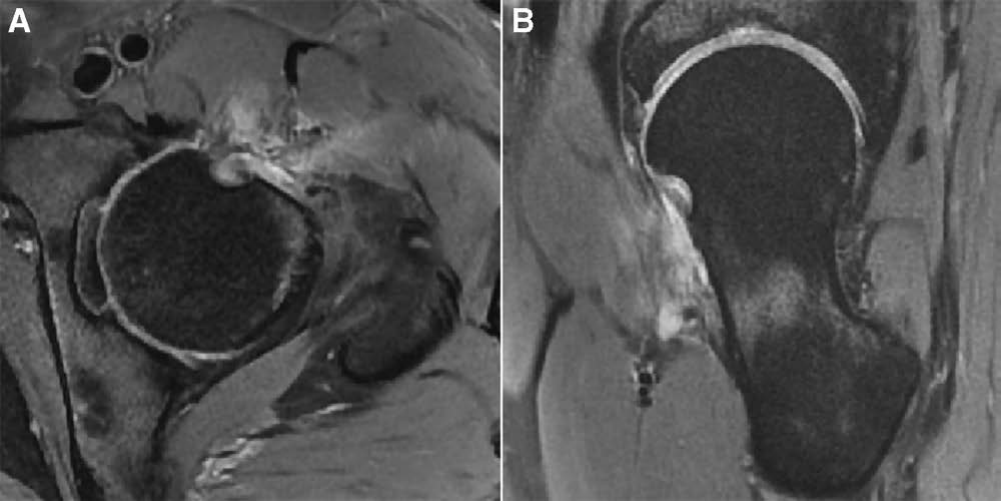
(A, B) Axial and oblique sagittal MRI 6 months after surgery showed no local recurrence. MRI = magnetic resonance image.
